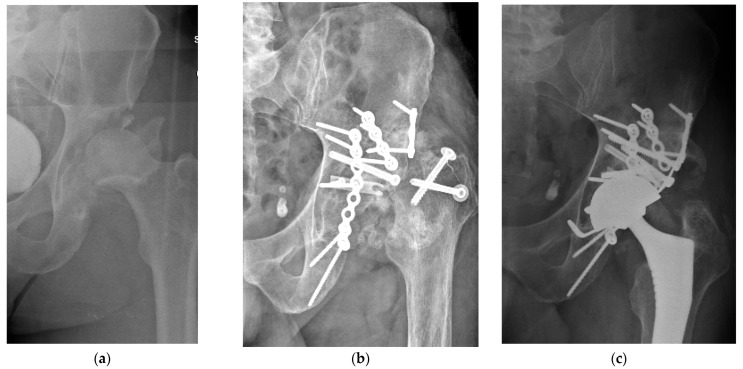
(a) Posterior hip dislocation with an associated posterior column fracture. (b) Seven-month follow-up showing femoral head osteonecrosis. (c) Postoperative radiograph after total hip arthroplasty.
